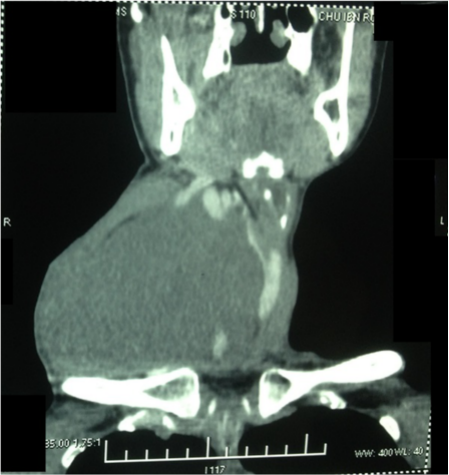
Coronal computed tomographic scan showing the patient’s cervical mass, which was huge, well-defined, measured 110 × 100 × 147 mm, and occupied the right carotid triangle
